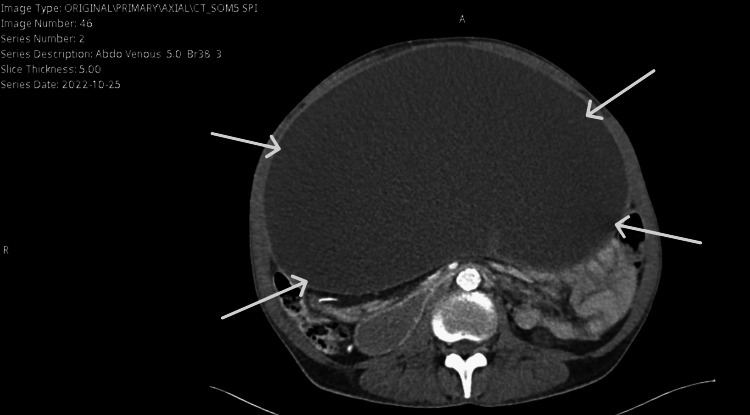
CT Abdomen with contrast, cross-sectional view White arrows point to a large anterior intra-abdominal cystic lesion occupying the entire anterior abdomen.
